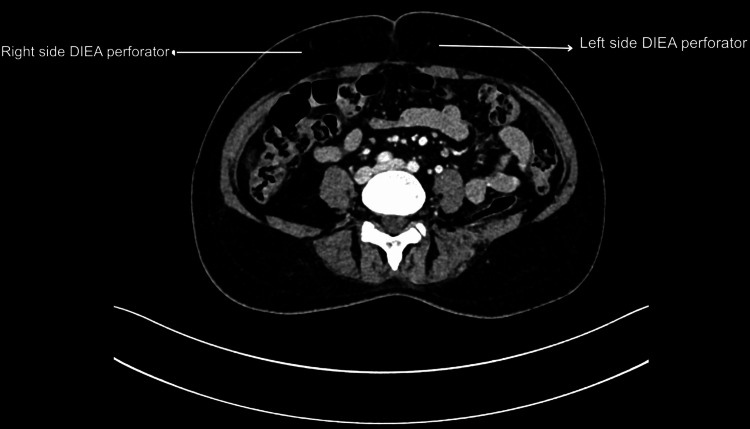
Smaller diameter of deep inferior epigastric perforators bilaterally on CT Imaging DIEA: Deep inferior epigastric artery
